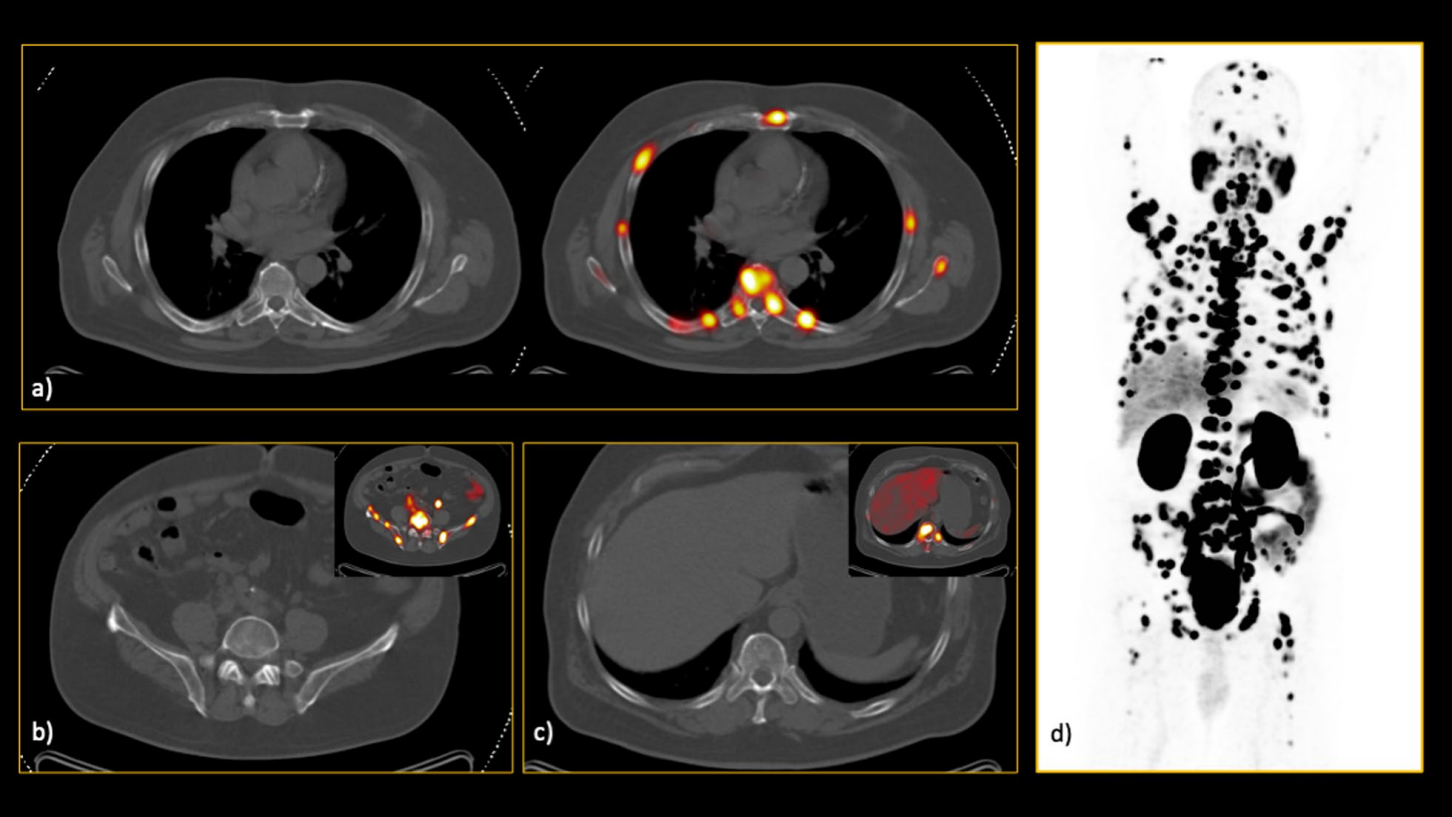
In this interesting case, a 66-year-old man referred for initial staging, there was extensive involvement of both axial and appendicular skeleton. However, none of the lesions had significant characteristics on the low-dose CT images, resulting in calling this patient free of skeletal involvement in CT evaluation by all readers. As you can see in the hybrid ^68^Ga-PSMA PET/CT images, there were innumerable bone metastases which all were invisible on CT alone (a–c). d The maximum intensity projection (MIP) clearly visualises the extent of the disease
